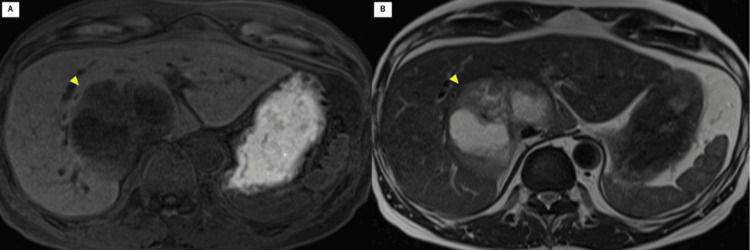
Magnetic resonance imaging. (A) T1-weighted magnetic resonance (MR) image showing a tumor with low signal intensity. (B) T2-weighted MR image showing low signal intensity at the margin and high signal intensity at the center of the tumor.
